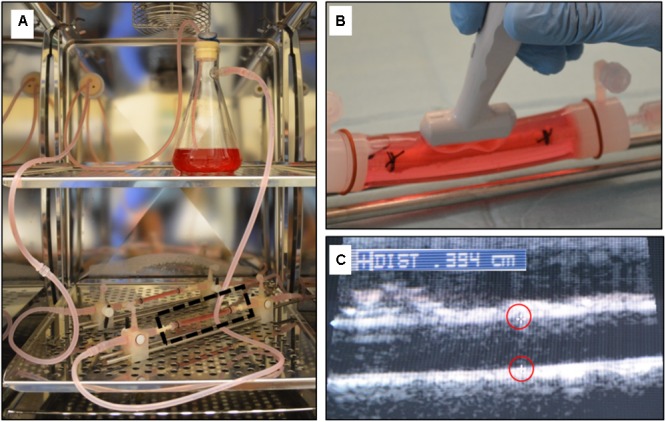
Ex vivo* bioreactor system. (A) A gear pump generated pulsatile through explanted arteries within the CO2 incubator. (B) The explanted artery is bathed in cell culture media and an ultrasound probe can be directly placed on the outer sleeve of the bioreactor housing to measure the diameter. (C) Ultrasound image showing the inner diameter of the explanted artery (markers outlined in red).
